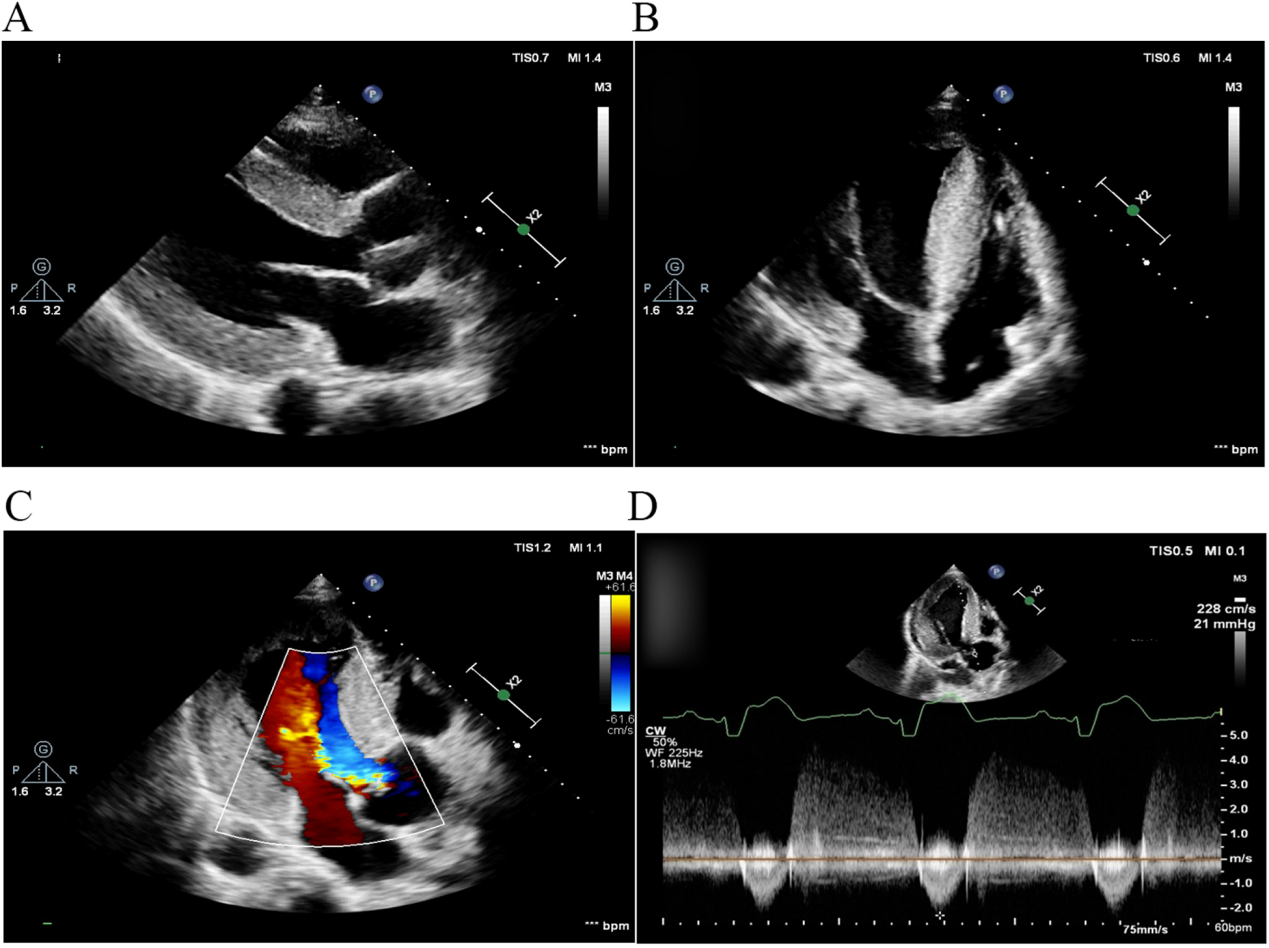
TTE 1 month after left ventricular outflow tract dredging. (A) Parasternal long-axis view of the left ventricle and (B) apical four-chamber view. The diastolic interventricular septal thickness decreased to 22 mm. (C) Color Doppler flow imaging and (D) continuous wave Doppler demonstrated that left ventricular outflow tract obstruction was alleviated, with a peak gradient reduced to 21 mmHg. TTE, transthoracic echocardiography.
